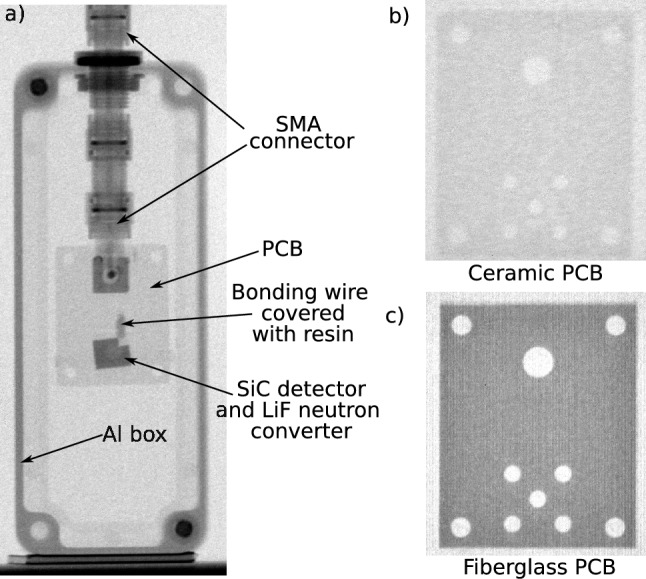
(a) Neutron radiography of the detector mounted on a ceramic PCB in an aluminum box using the standard STORNI facility. Neutron radiograph of a ceramic PCB (b), and fiberglass PCB (c).
